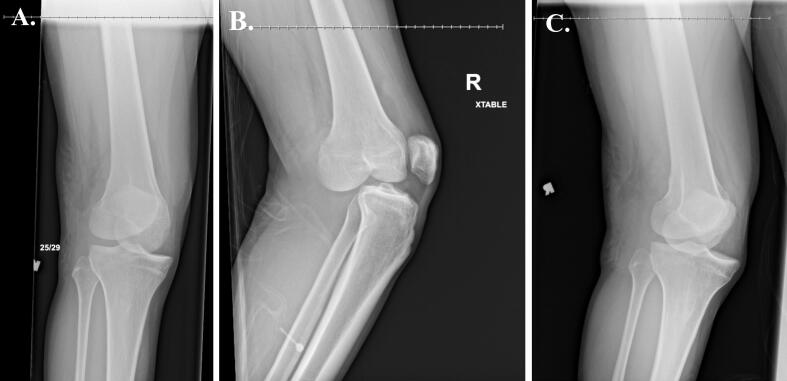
Radiographs of the right knee demonstrating (A) AP, (B) lateral, and (C) oblique views of the right knee dislocation at initial presentation demonstrating an anterior dislocation.
